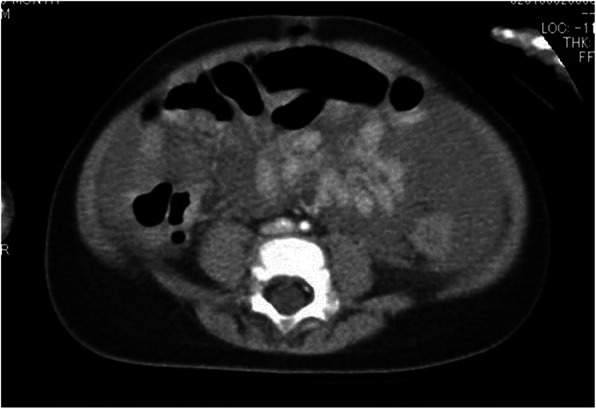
Initial computed tomography (CT), case 2. CT shows massive bloody ascites
